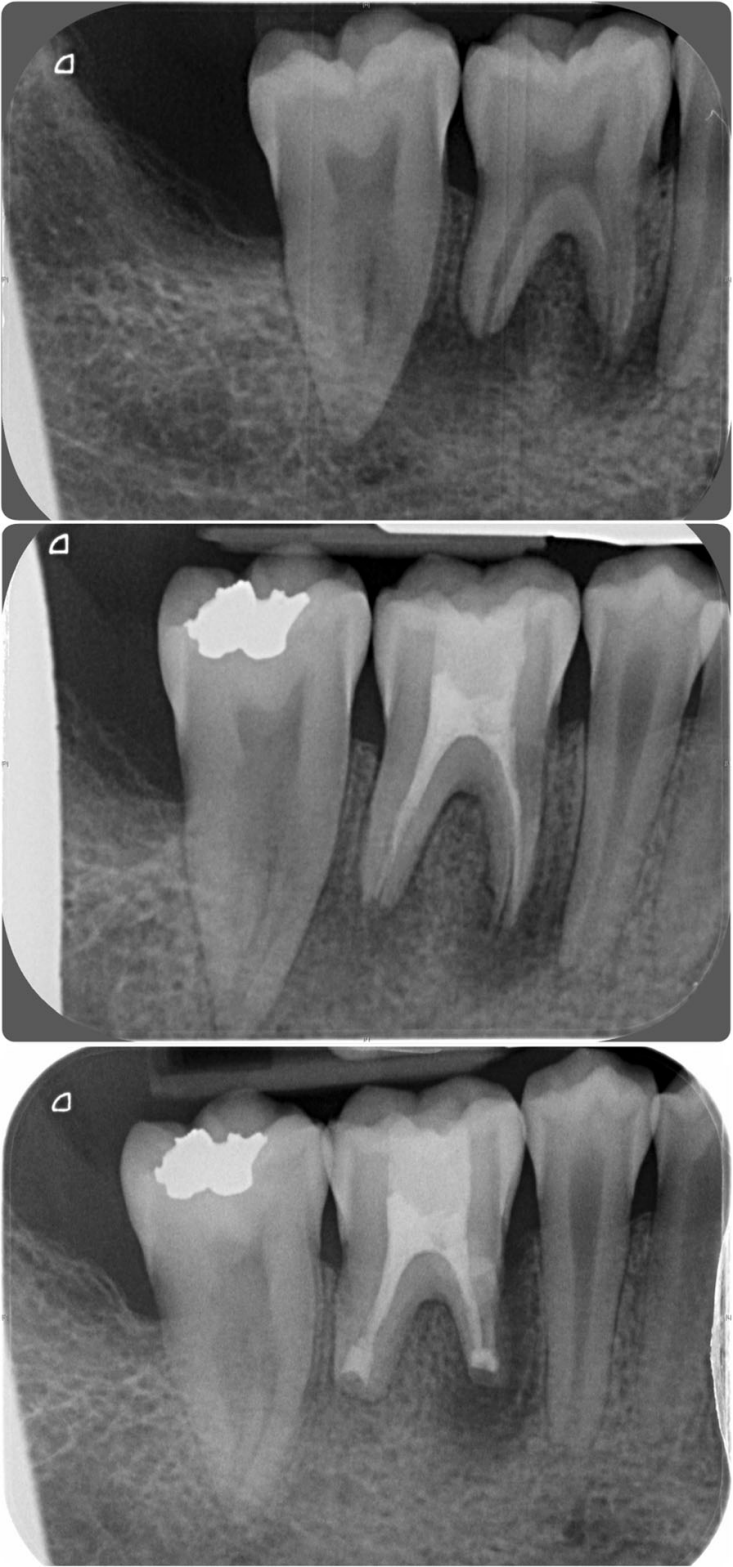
Failed endodontic treatments. Above, resorption associated with 46 and 47 and a periapical radiolucency associated with 46; middle, attempted root canal therapy of tooth 46; below, attempted intentional reimplantation with extra-oral root end surgery and retrograde root filling of tooth 46
